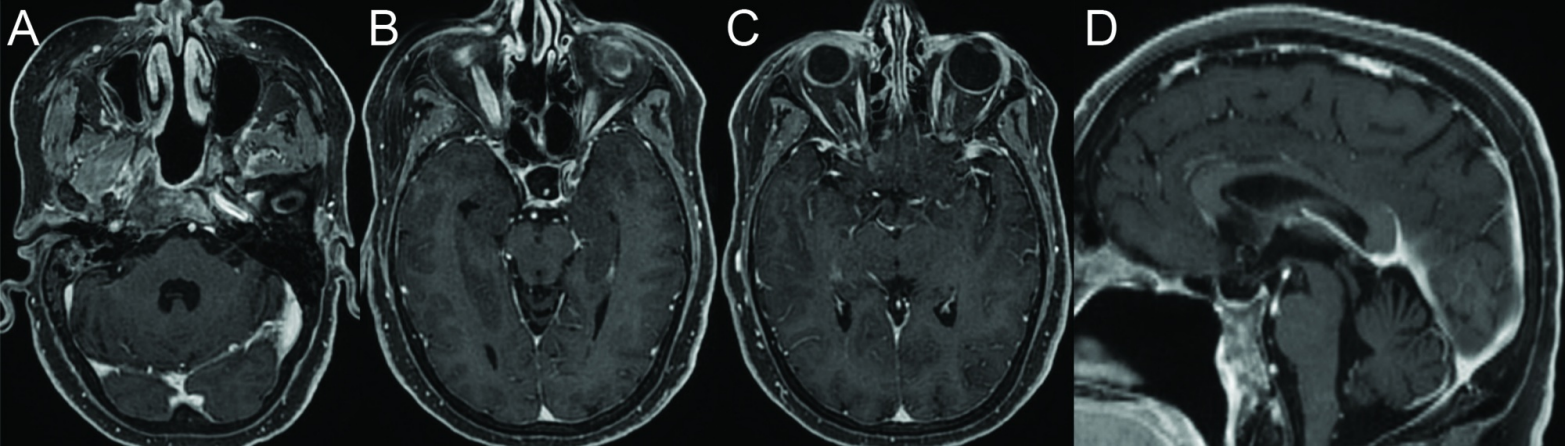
Postoperative MRI after resection of skull base chondrosarcoma. Axial T1-weighted MRI with contrast shows near total resection of the chondrosarcoma two years after surgical resection (A, B, and C). Sagittal MRI shows dramatic reduction in tumor size and alleviation of brainstem compression (D).
